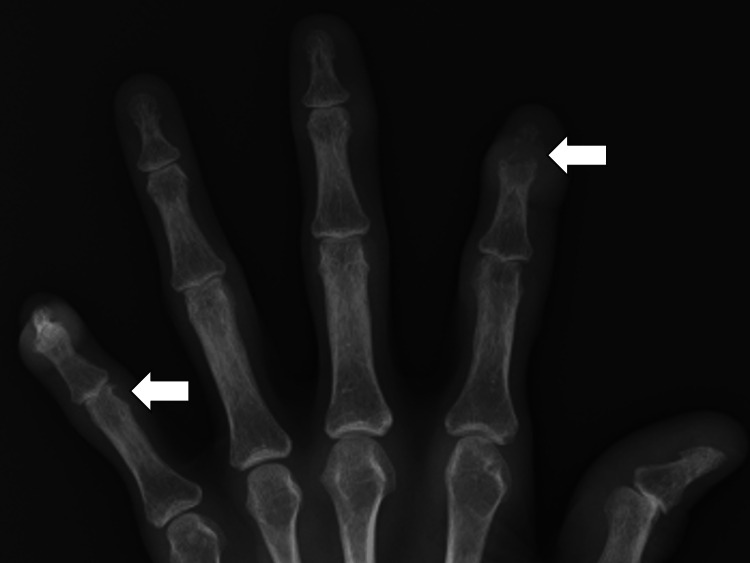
Radiographs of the fingers showing overhanging edges in the joints (white arrows).
